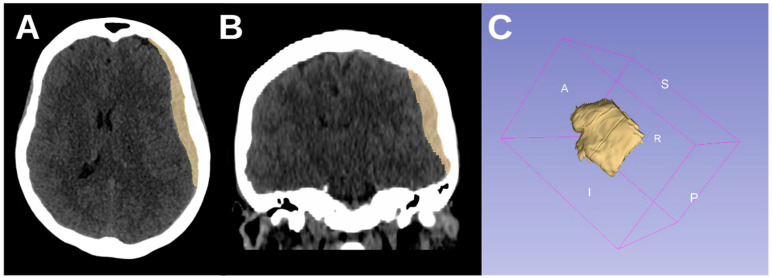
CT axial (A), coronal (B), and 3D reconstruction (C) of an acute subdural hematoma with lower elongation (1.38206) in a 68-year-old female patient with generalized seizures.
